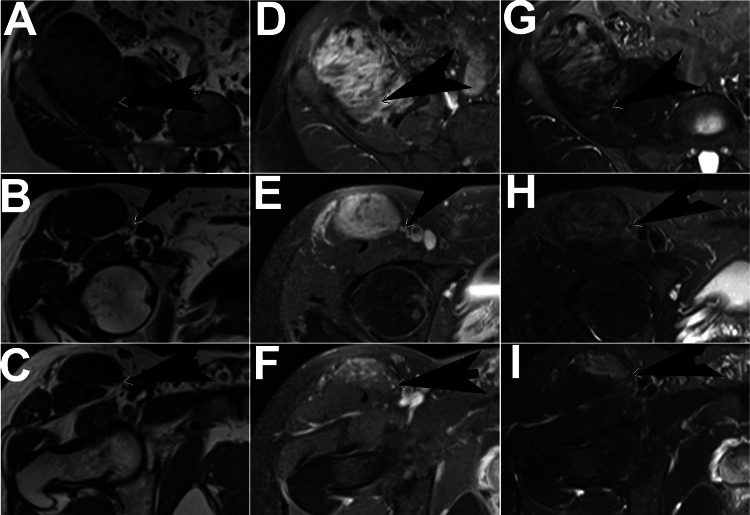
Sequential axial MR images of Case 2 Left column (A-C): T1 sequence; middle column (D-F): T1 fat saturation sequence post contrast; right column (G-I): T2 fat saturation. Black arrows indicate nerves: in the top row, the femoral cutaneous nerve (A, D, G) and in the second (B, E, H) and third rows (C, F, I), the femoral nerve
